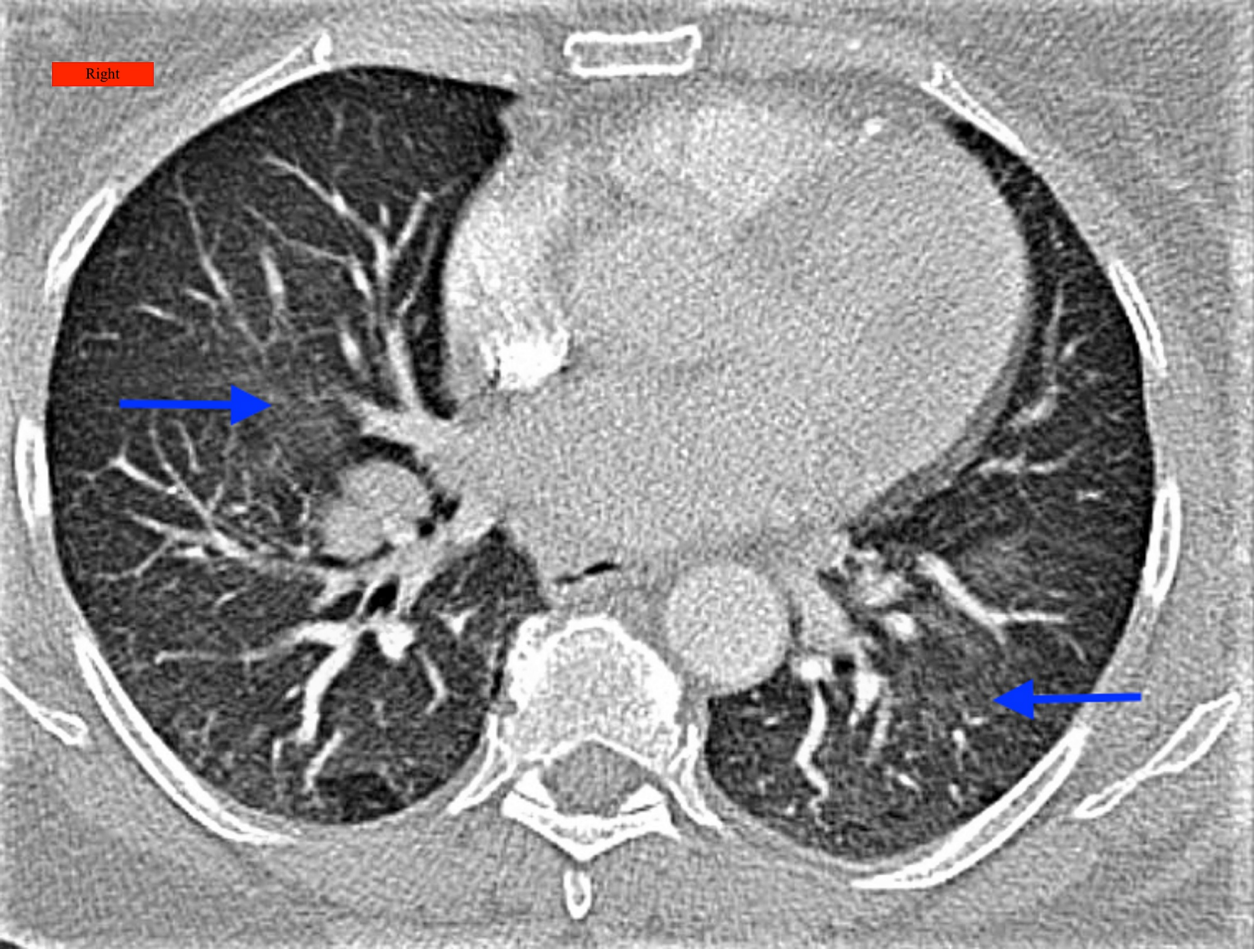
CT chest showed minimal scarring with mild ground-glass opacities in the bilateral lower lobes (arrows) Right: Indicates the right side of the patient; CT: Computed Tomography
